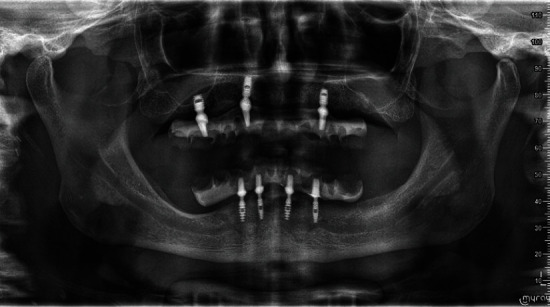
Postoperative orthopantomography at the 2-year follow-up.
